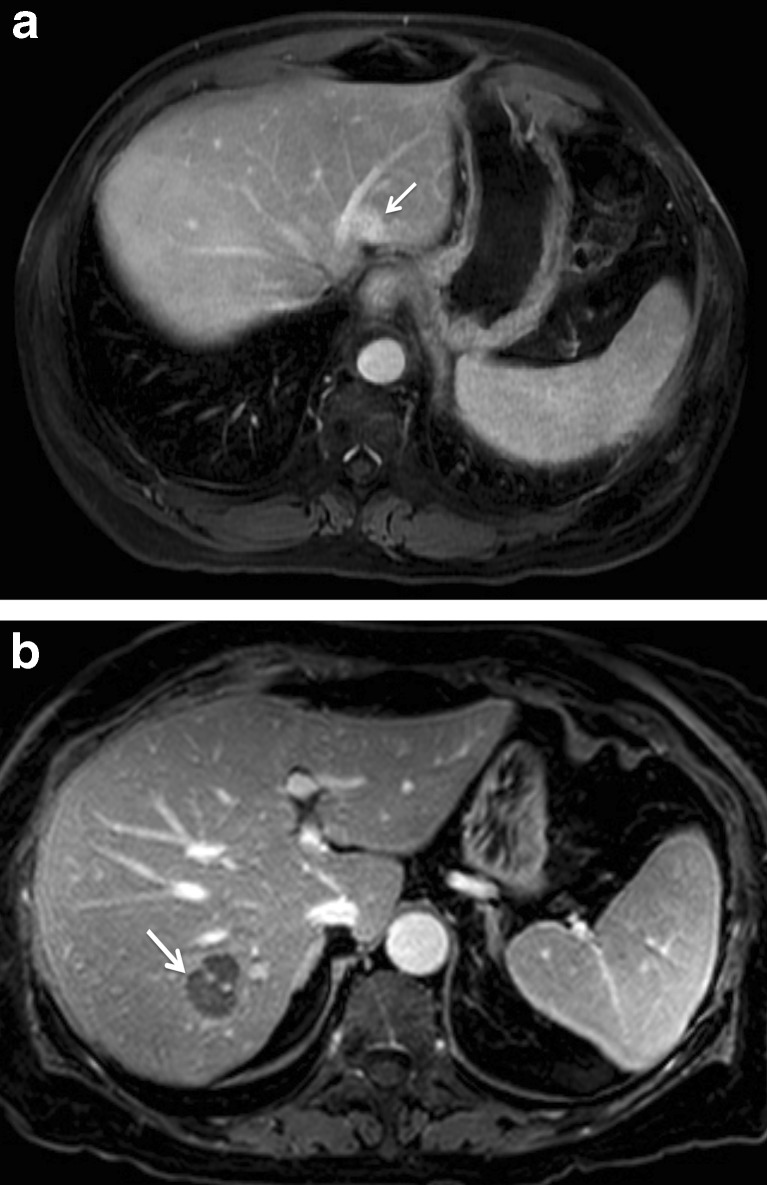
Colorectal liver metastases seen on 10-min delayed-phase, gadobutrol-enhanced MRI (a) in a 75-year-old male with strong target tumour enhancement and (b) in a 60-year-old male with weak target tumour enhancement
